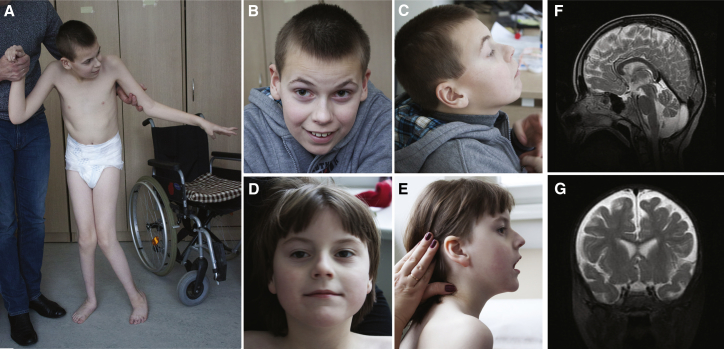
Pictures and Brain MRI from Surviving Individuals Front and side views of the LT affected brother LT.II.1 (A–C) and sister LT.II.2 (D, E) at the ages of 13 years and 7 years, respectively. Brain MRI images of affected individual LT.II.1 at age of 8 years showed small posterior fossa arachnoid cyst, discrete vermian atrophy, and slight increase of the fluid-filled retro and infra-cerebellar space (F). Brain MRI images of affected individual LT.II.2 at age of 1 year showed discrete parenchymal rarefaction involving mainly the frontal lobes (G).
